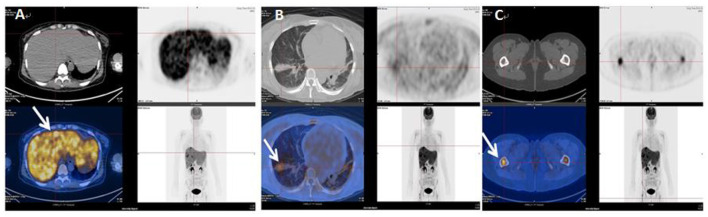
Whole body PCT-CT examination on January 8, 2020. (A) indicates hyper-metabolic lesions (white arrows) in the liver. (B) indicates hyper-metabolic lesions (white arrows) in the lungs. (C) indicates hyper-metabolic lesions (white arrows) in the femur.
